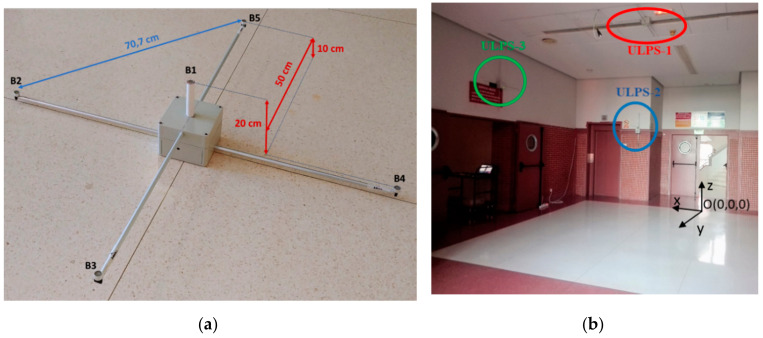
(a) General view of the LOCATE-US 3D ultrasonic local positioning system (ULPS) developed by the GEINTRA-US/RF research group from the University of Alcala [11]; (b) Configuration proposed for the 3D positioning system, based on three ULPSs installed on three perpendicular planes in the experimental environment, where the point O is the origin of coordinates.
